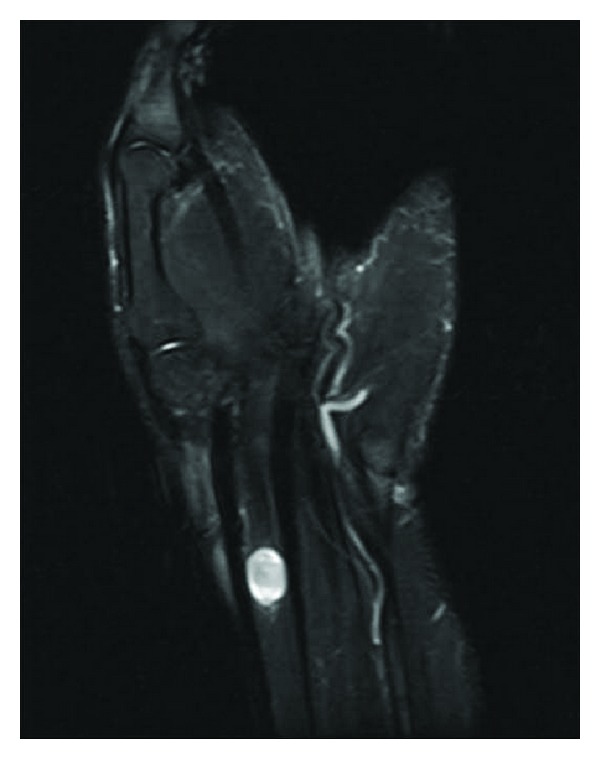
MRI revealed a 11 × 9 mm mass located in flexor tendons which has intermediate signal on T1-weighted images and hyperintense signal on T2-fat weighted images.
